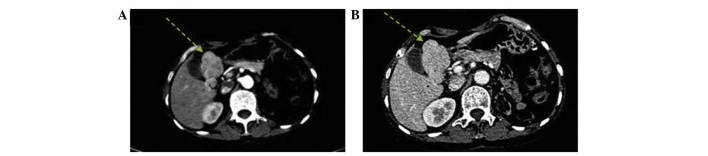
CT imaging of the abdomen. CT imaging in the (A) arterial and (B) portal phases revealed no spleen and a maximum diameter of 6.1×3.6 cm mass (arrows) arising from the duodenal bulb, mimicking a duodenal tumor.
